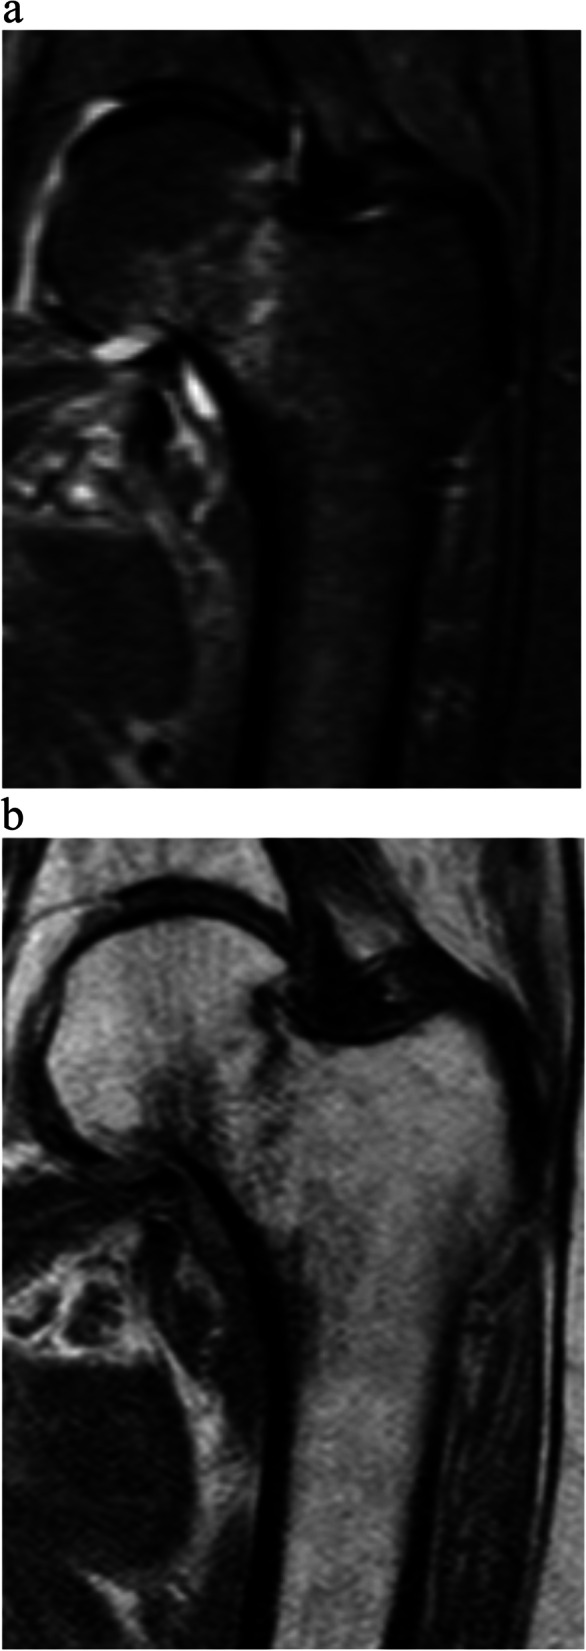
MRI of the same occult femoral neck fracture. a) T2 weighted anteroposterior image. b) T1 weighted anteroposterior image
